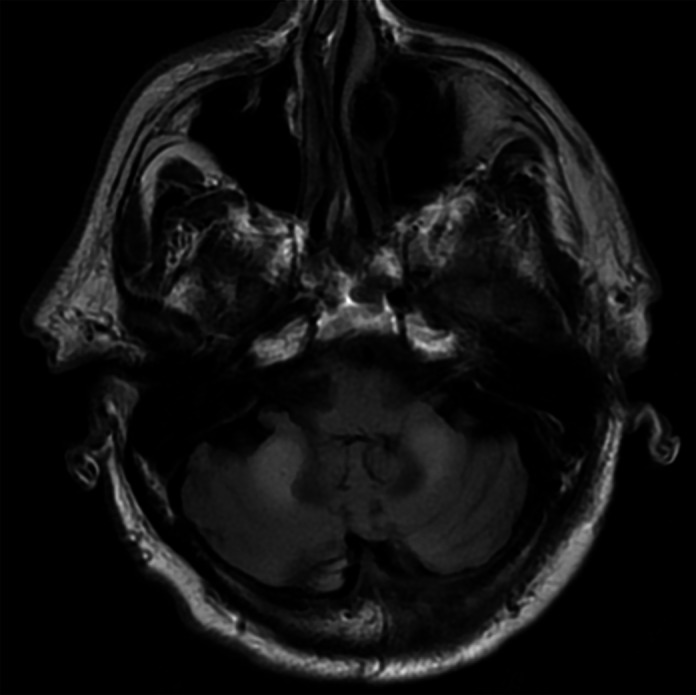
Magnetic Resonance Imaging on FLAIR sequence demonstrates symmetric FLAIR signal abnormality in the cerebellar peduncles bilaterally.
